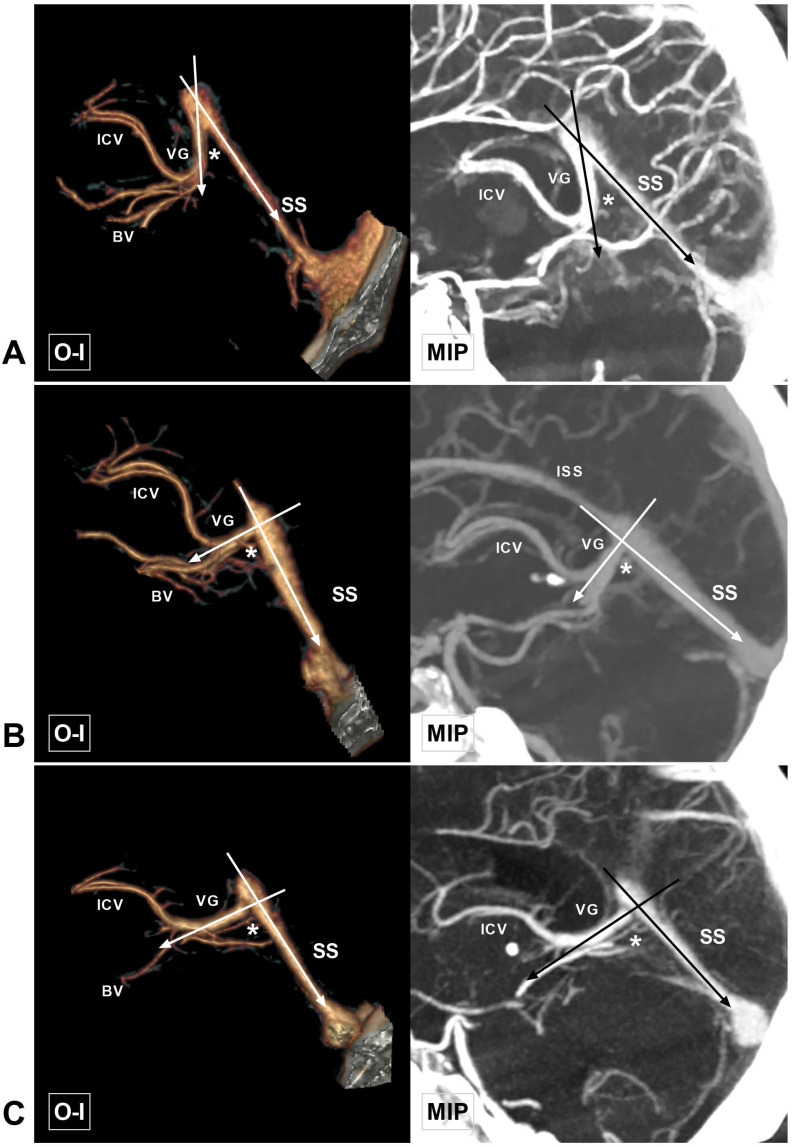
Different angles at the VG and SS transition area. A, CTA and MIP show an acute angle at the VG and SS transition area (asterisk). B, CTA and MIP show a right angle at the VG and SS transition area (asterisk). C, CTA and MIP show an obtuse angle at the VG and SS transition area (asterisk). Abbreviations: BV, basal vein; CTA, computed tomography angiography; ICV, internal cerebral vein; ISS, inferior sagittal sinus; MIP, maximum intensity projection; O-I, outside to inside; SS, straight sinus; VG, vein of Galen.
